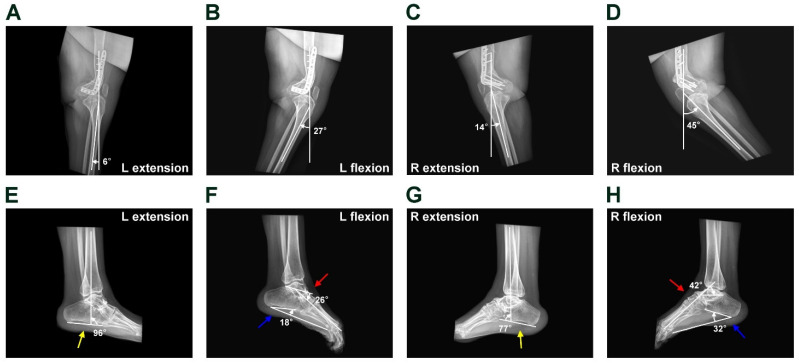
Postoperative 3-year lateral radiograph of both knees and ankle-foot dynamic films. The views show the flexion and extension angles in the left and right knees (A–D). The dynamic lateral films of the foot/ankle show the tibiocalcaneal angle (yellow arrow), Meary’s angle (red arrow) and Calcaneal pitch (blue arrow, (E–H)).
